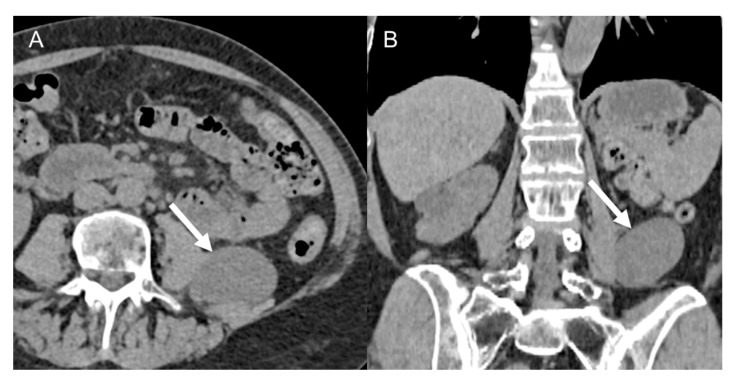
Retroperitoneal schwannoma in 66-year-old woman. Axial (A) and coronal (B) non-contrast CT images show 5.4 cm homogeneously hypodense lesions with posteriorly to the right psoas muscle (arrow).
